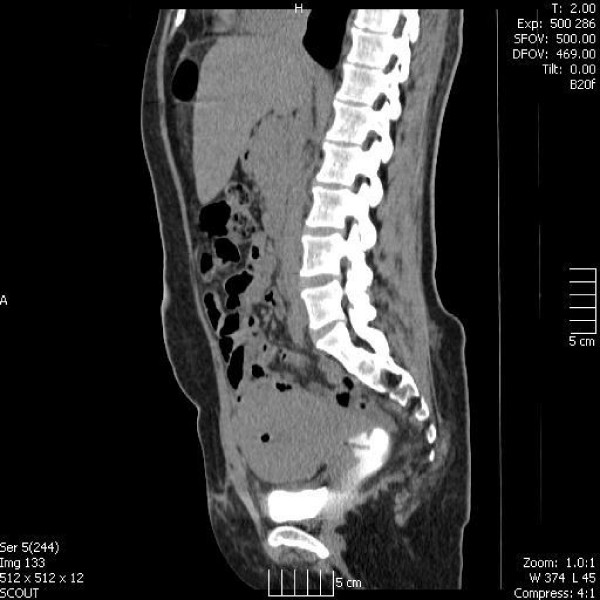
CT-IVU showing contrast in the right ureter draining into the uterus.
